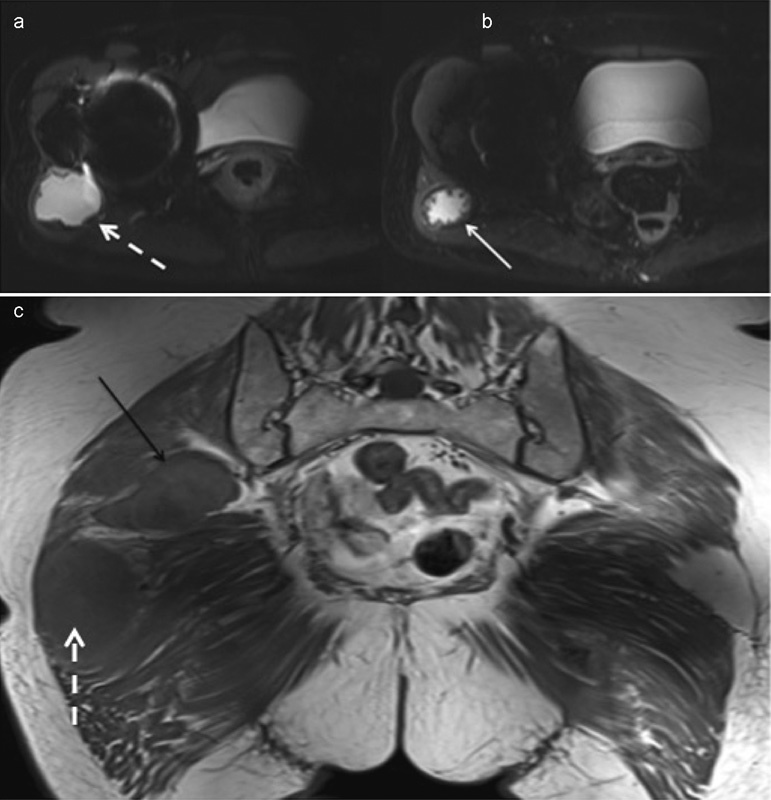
Axial short tau inversion recovery magnetic resonance imaging (MRI) confirms a periarticular cystic structure related to the right hip joint (dashed white arrow in a and c) with a low signal pseudocapsule (solid white arrow in b) in keeping with an adverse local tissue reaction (ALTR). The ALTR (dashed white arrow) is isointense to skeletal muscle on coronal spin-echo T1 sequence (c). There is a second discrete lesion (solid black arrow in c) within the gluteal muscle with a rim that is isointense to muscle and intralesional hypointensity suggestive of turbulent flow in keeping with a pseudoaneurysm.
